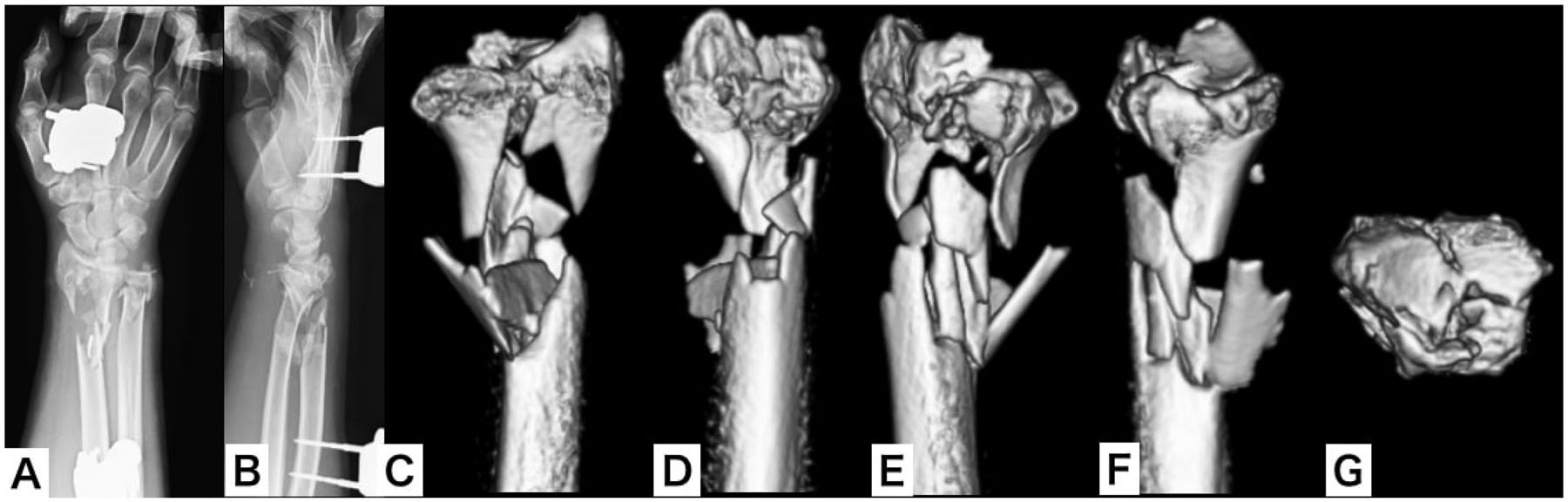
Plain x-ray and three-dimensional computed tomography of the right wrist after external fixation as a first-line treatment. (A,B) Plain x-ray of the right wrist; (C–G) 3-D CT of the right wrist.
